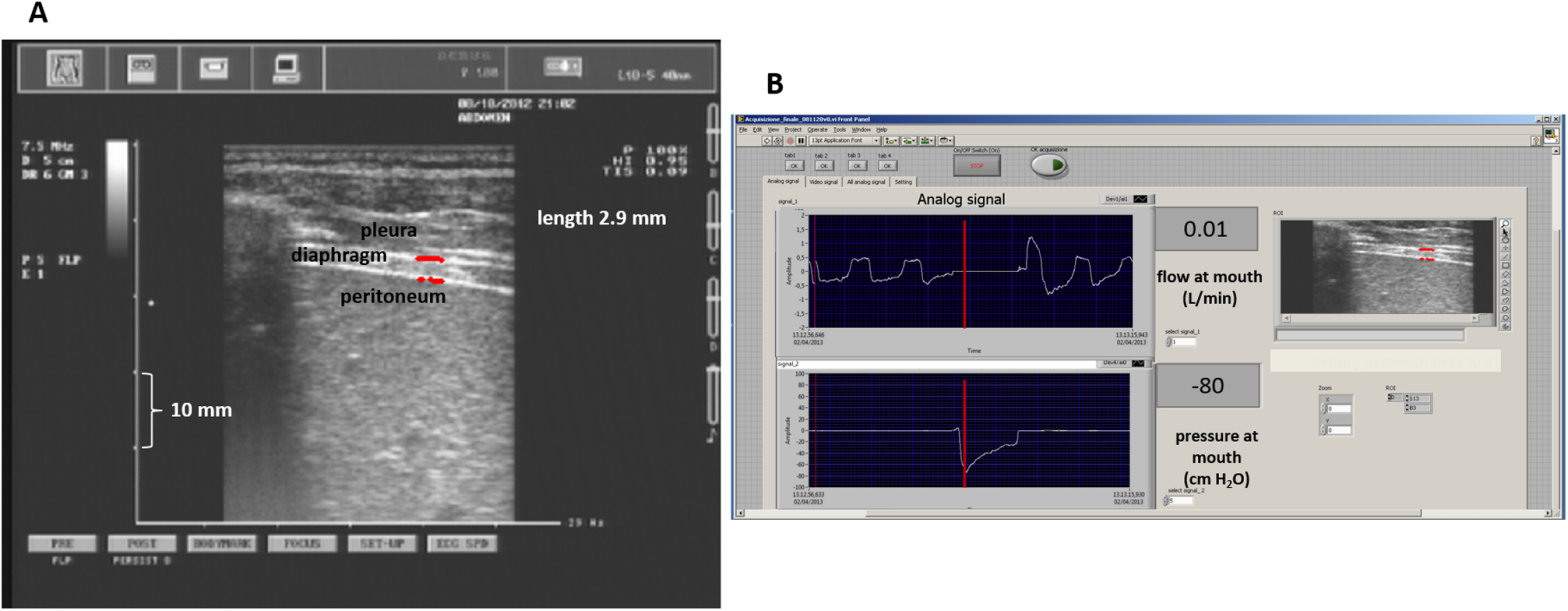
Diaphragm B-mode ultrasound and acquisition software front panel. (A) Diaphragm thickness (DT) at maximal inspiratory pressure maneuver in supine position measured as the distance between pleural and peritoneal membranes of the diaphragm colored in red; (B) front panel of the Labview software for the synchronously recording of ultrasonographic images and flow and pressure signals.
